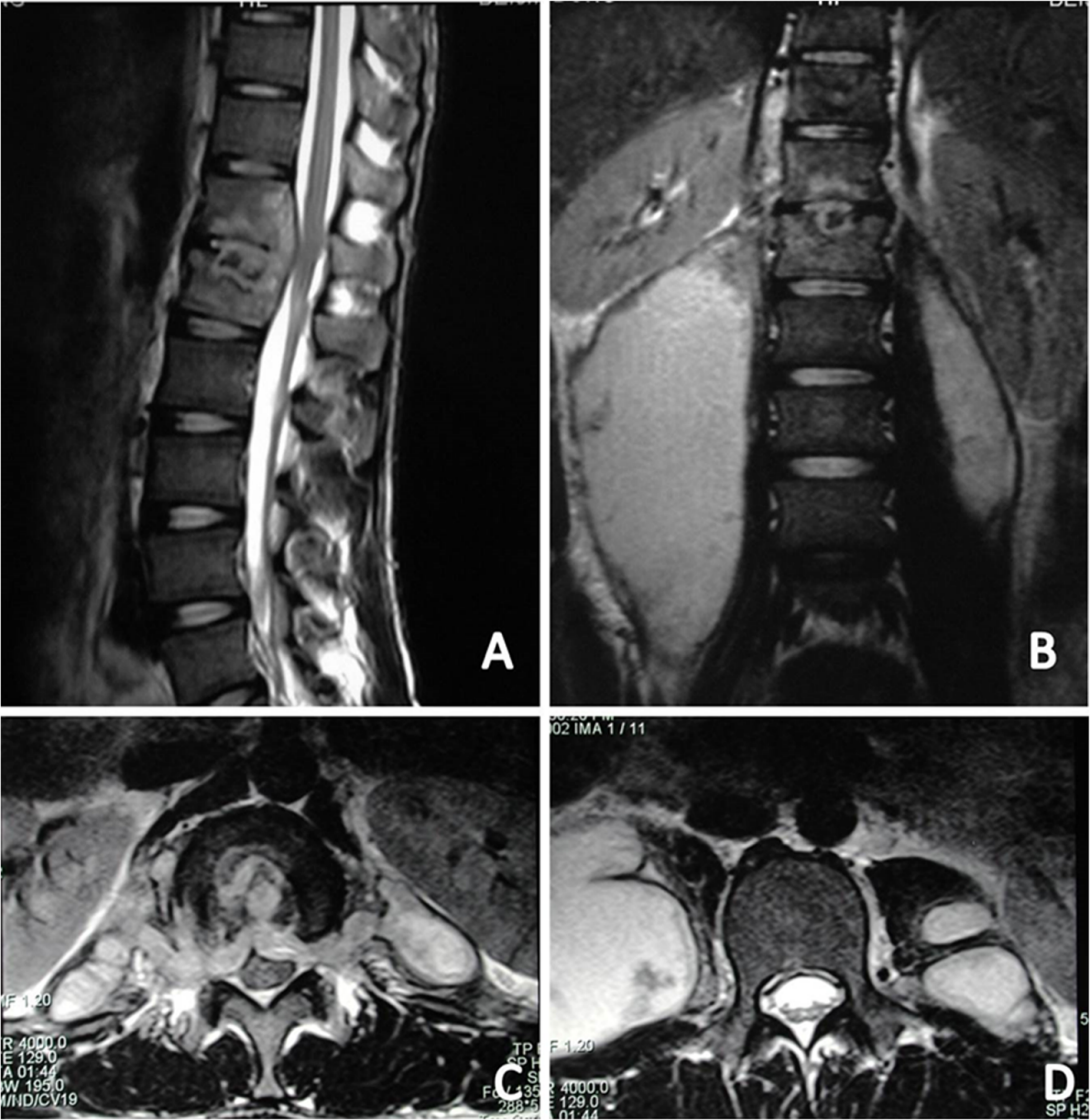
MRI showed that both sides of psoas abscess existed in a 22-year-old male patient with tuberculous spondylitis of T12-L1. a Sagittal T2-weighted MRI demonstrated a T12-L1 infection source and associated bilateral psoas muscle abscess. b Coronal T2-weighted magnetic resonance imaging (MRI) demonstrated that the right kidney was not in the right place because of the right huge psoas abscess. c and d showed the para-vertebral abscess and psoas abscess existed in the relative section of the axial section
